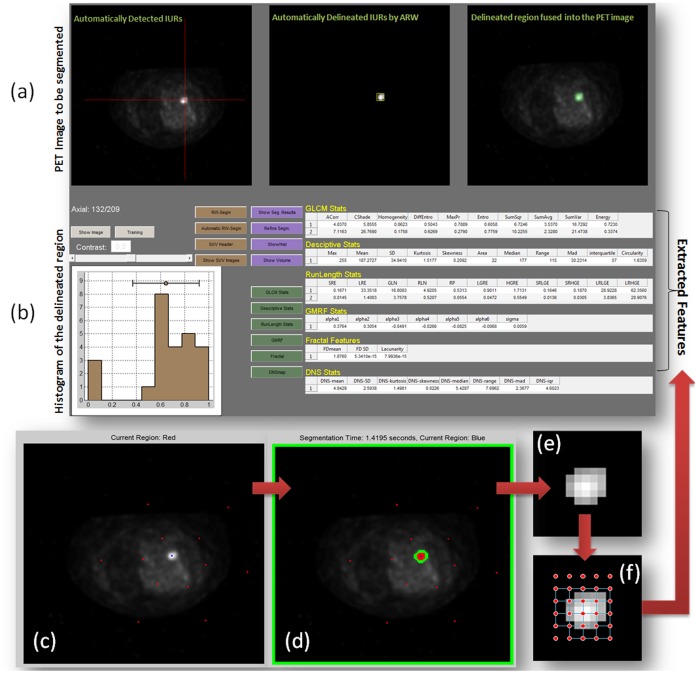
An example view from our proposed framework/software is shown. (a) Automatically detected uptake regions (first), its segmented version (second), and fusion of segmented region into original image (third) are shown. After detection, the details of object (blue) and background (red) seeding and conducted segmentation are shown in (c) and (d), respectively. Segmented region (e) is divided into local windows and for each local window pre-defined textural and shape features are extracted (f). Tools to control extraction of textural features, segmentation, SUV analysis, and the immediate results are shown in (b).
